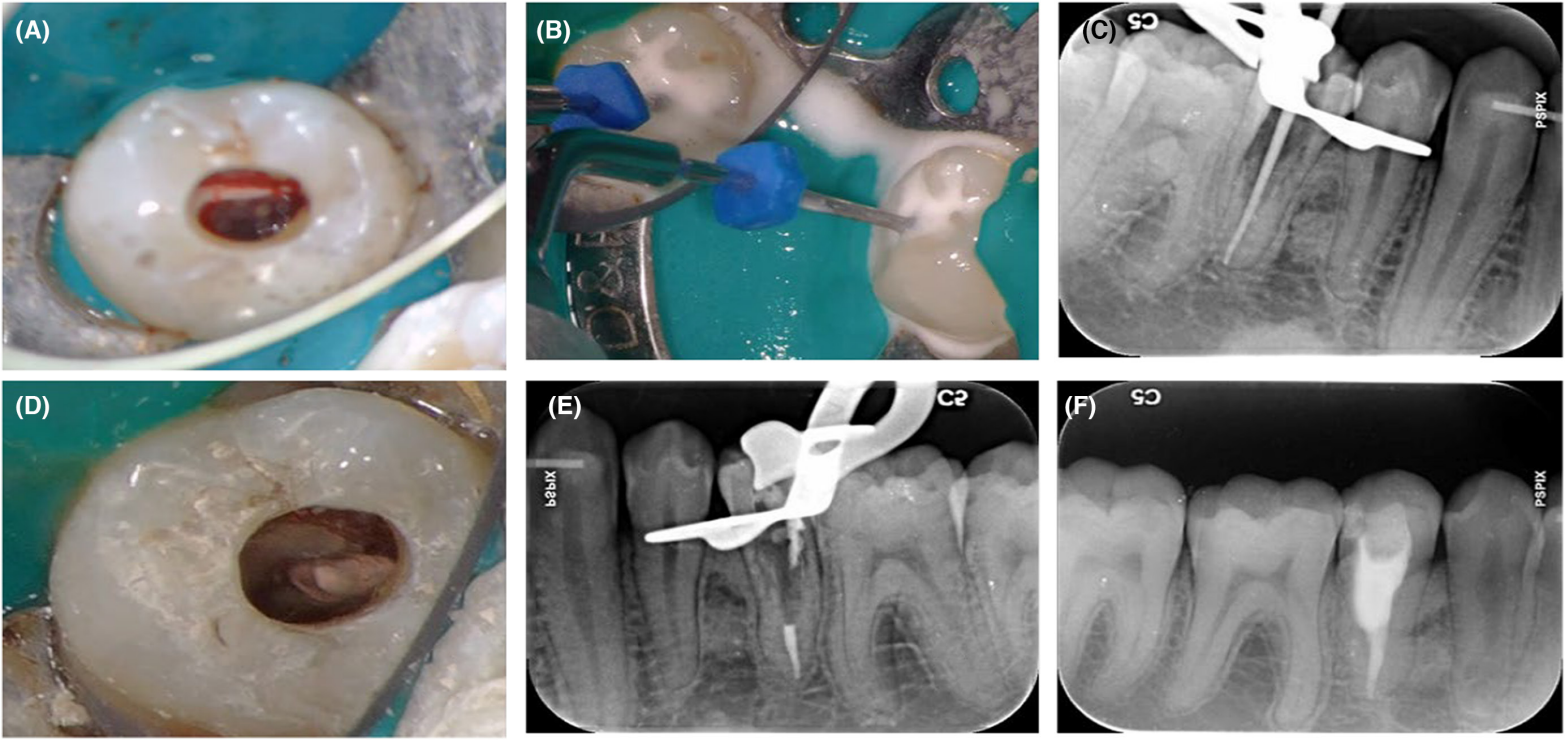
(A) Conservative access cavity preparation. (B) Sodium hypochlorite solution and its activation with ultrasonic. (C) Confirming MAC by radiography. (D) Cutting off the gutta percha at the 3 mm area from the apex under DOM. (E) Cutting off the gutta percha at the 3 mm area from the apex. (F) The remaining portion of the canal was filled with cold ceramic.
